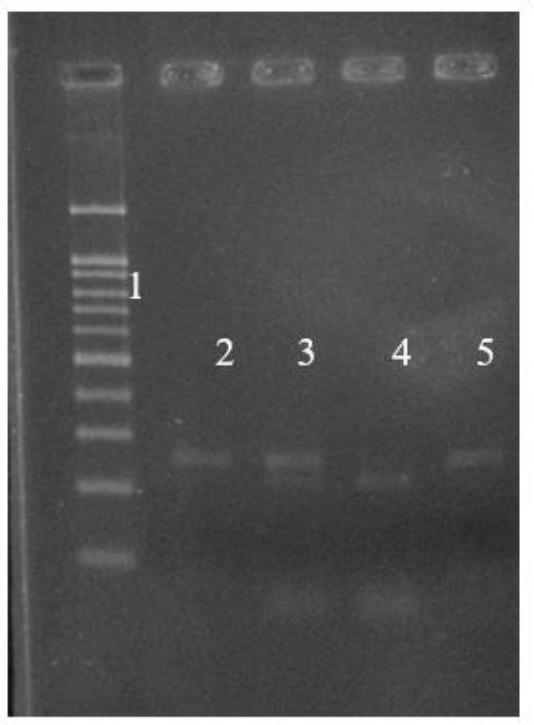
Analysis of the T786 polymorphism of the eNOS gene. Lane 1: 100 base pair (bp) DNA Ladder, Lane 2, 5 TT genotype, Lane 3 :CT genotype, Lane 4 CC genotype
